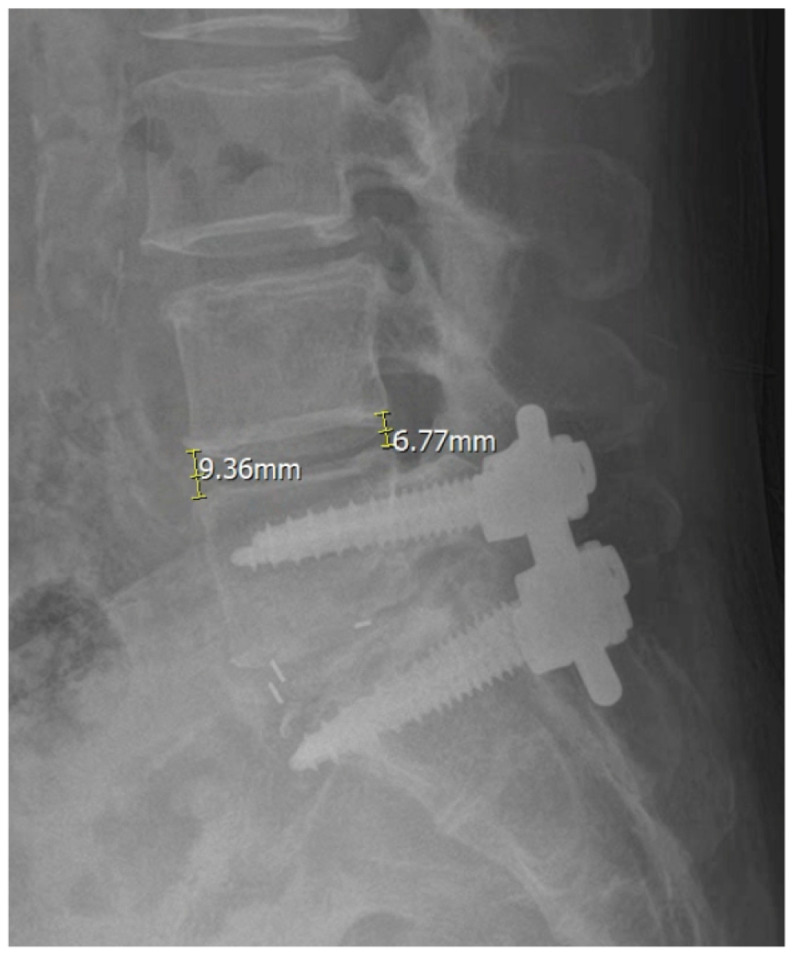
Measurement of anterior disk height (ADH) and posterior disk height (PDH) on lateral radiographs.
